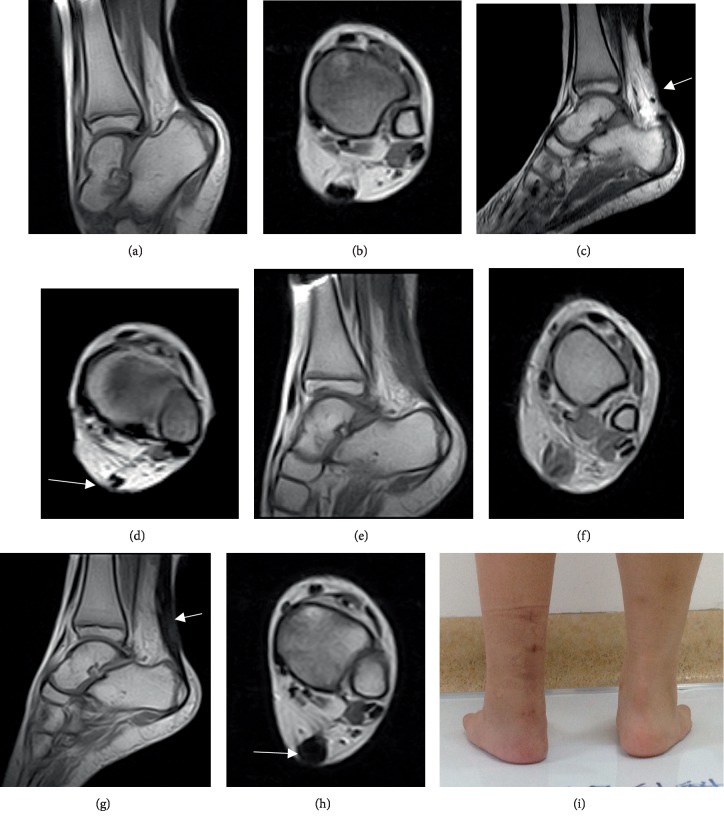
MRI images and photo of a 8-year-old female. Preoperative MRI showed Achilles tendon and equinus (a, b). MRI showed Achilles tendon was transected over half of the tendon while tendon was not totally torn (arrowed site) after 2 days of percutaneous Achilles tendon lengthening (c, d). Wounds in tendon were healed at 2 months postoperatively (e, f). In the postoperative MRI images, Achilles tendon got wider (arrowed site) at 12 months postoperatively (g, h). After percutaneous Achilles tendon lengthening, the right equinus when standing disappeared at 2 months postoperatively (i).
